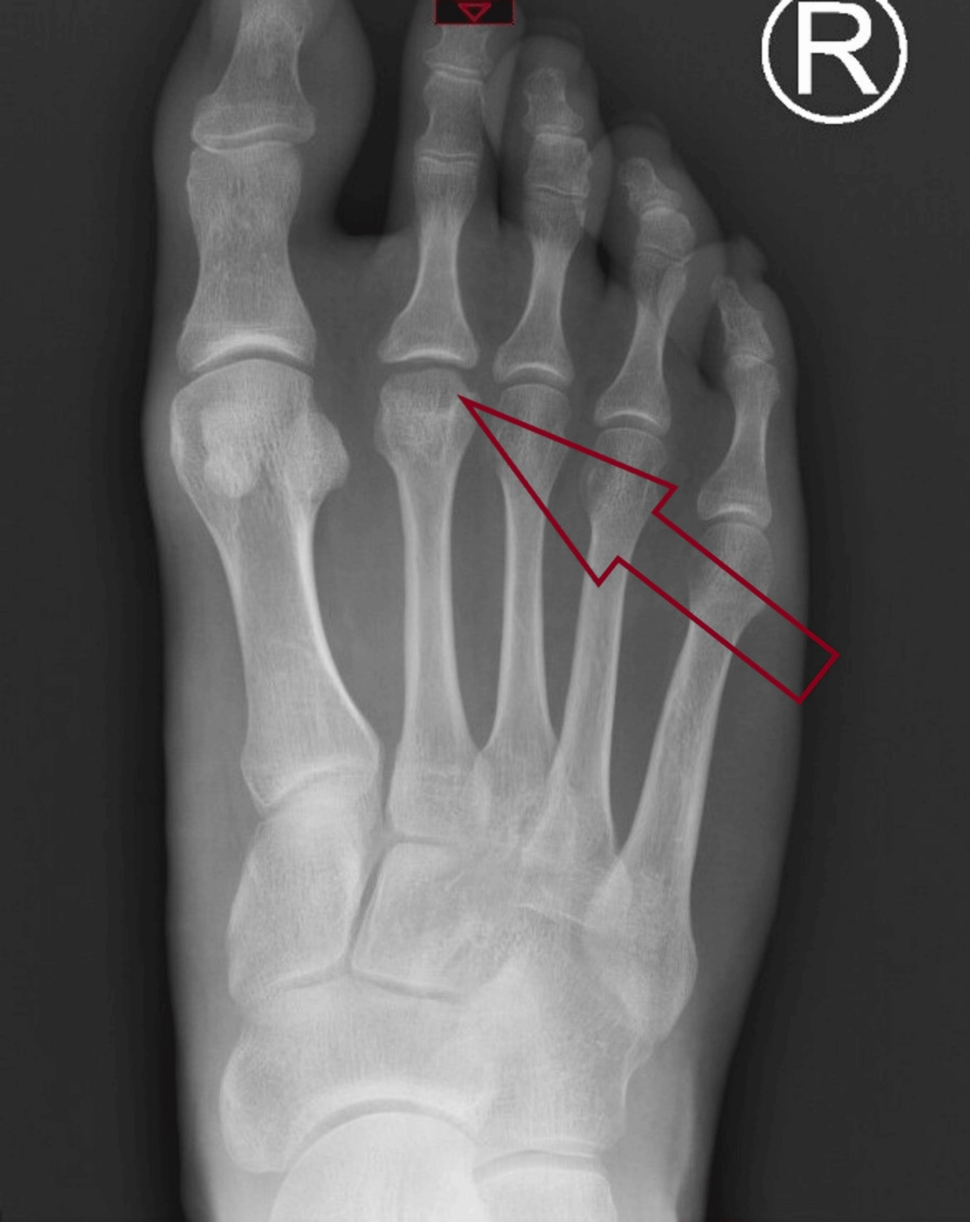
X-rays showed remodeling of the head of the right second metatarsal at the final follow-up.
